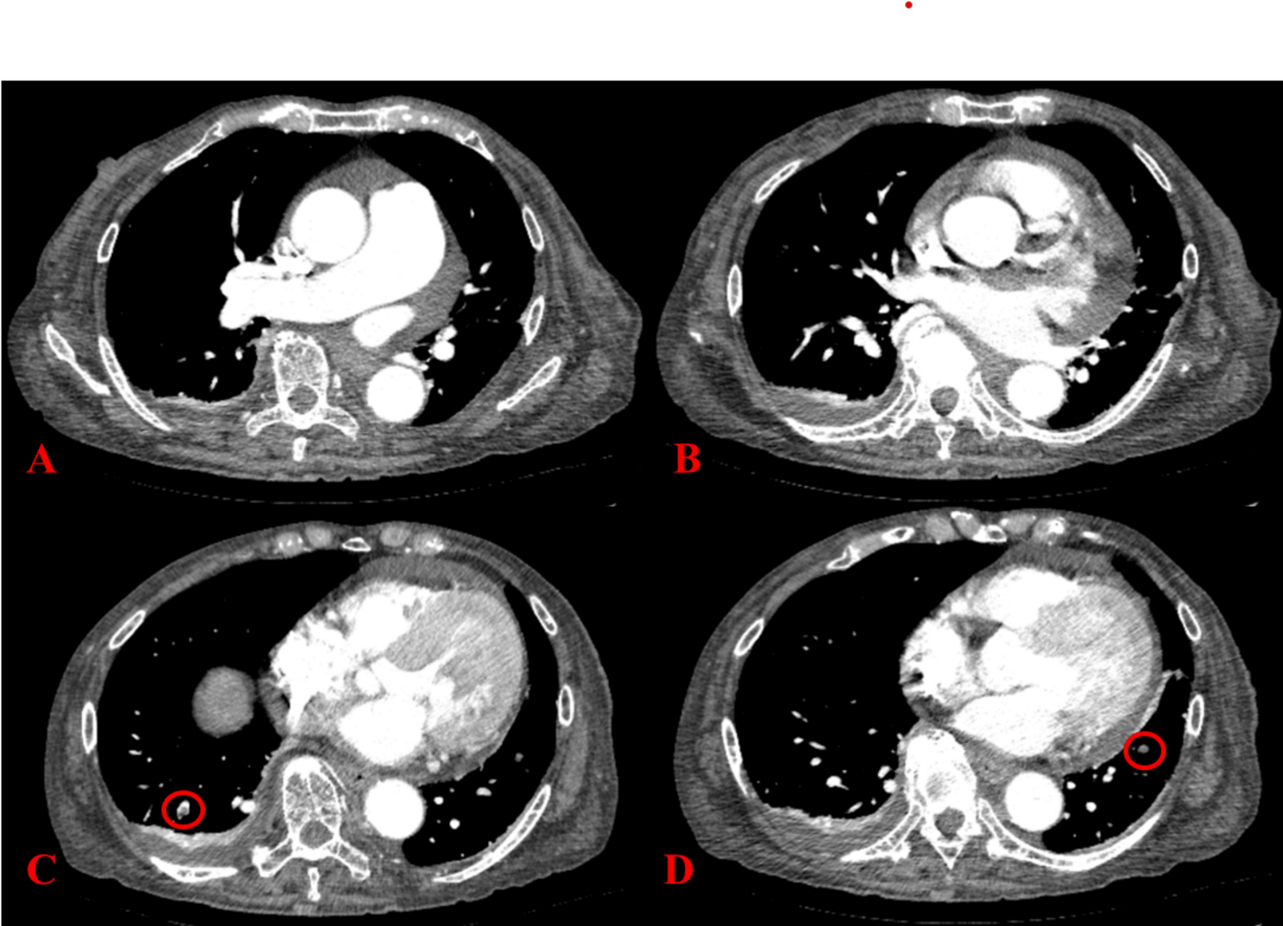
Findings of contrast-enhanced chest computed tomography Thrombi observed in the right (C) and left (D) basal pulmonary arteries. Because no thrombus was found in the main pulmonary arteries (A) (B) and no right ventricular dysfunction was identified, the diagnosis was low-risk pulmonary embolism.
